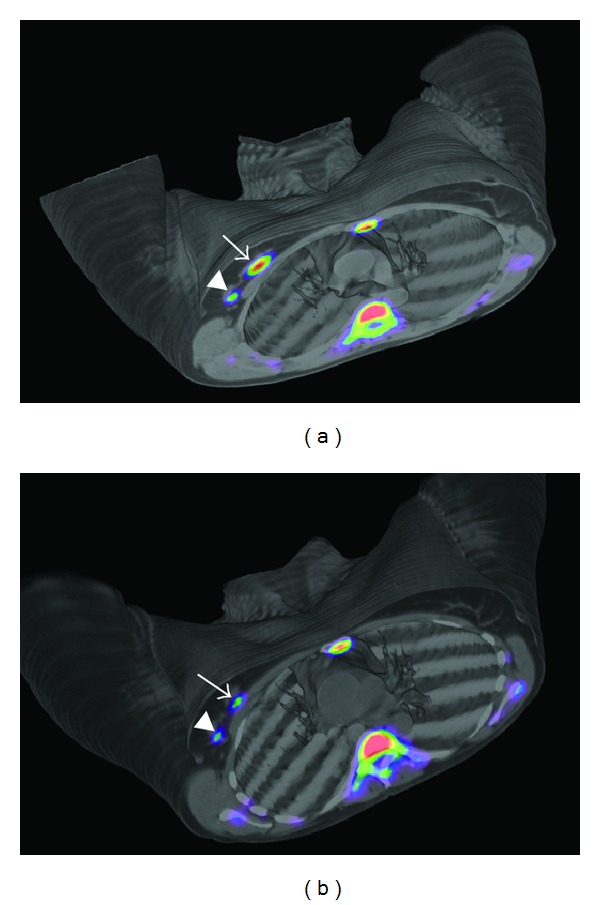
(a) Baseline PET/CT images obtained in a Biograph Duo LSO (Siemens) 75 min after injection of 405 MBq of ^18^FLT in a 47-year-old woman with a right-sided infiltrating ductal carcinoma (SUVmax = 5,42) (arrow) and lymph node uptake (SUVmax = 1,85) (arrowhead). Physiological bone marrow uptake was identified. (b) PET/CT images obtained 75 min after injection of 529 MBq of ^18^FLT after one cycle of neoadjuvant therapy. SUVmax decreased to 3,57 in the primary tumour and to 0,80 in the lymph node, consistent with metabolic response.
